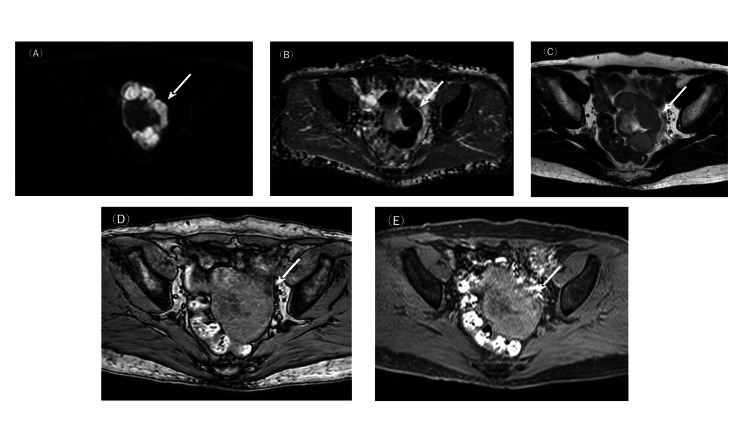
Axial magnetic resonance imaging of the pelvis The solid components showed high signal intensity on diffusion-weighted imaging (A), with low apparent diffusion coefficient values (B), corresponding to the mass on T2-weighted imaging (C). These components demonstrated low signal intensity on T1-weighted imaging (T1WI) (D) and pre-contrast fat-suppressed T1WI (E), with no evidence of fat or haemorrhage component.
